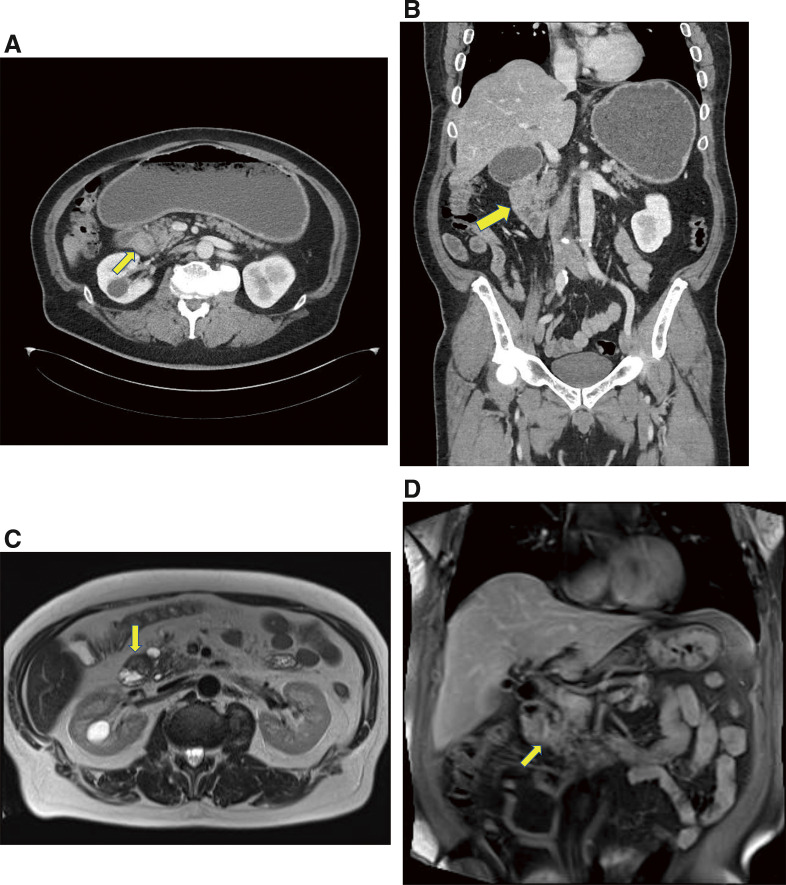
Computed tomography and magnetic resonance imaging findings at the first visit. (A and B) Computed tomography scan revealed duodenal obstruction and wall thickening in the second portion of the duodenum with stomach expansion, without lymph node swelling, and without dilation of the intrahepatic bile duct and main pancreatic duct (arrows). (C and D) Magnetic resonance imaging showed duodenal wall thickening (arrows).
